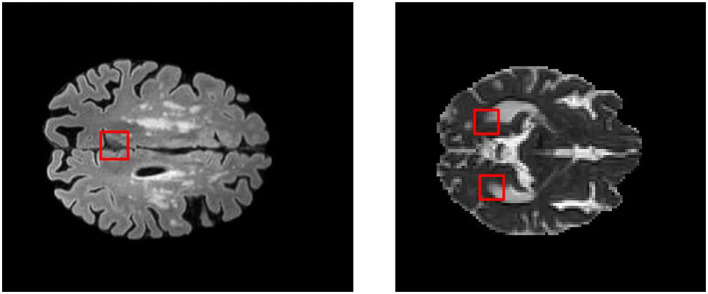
Examples of brain MRI images containing blurred lesion boundaries (highlighted by red rectangles). Such ambiguous regions are difficult to accurately delineate using conventional spatial-domain segmentation methods.
